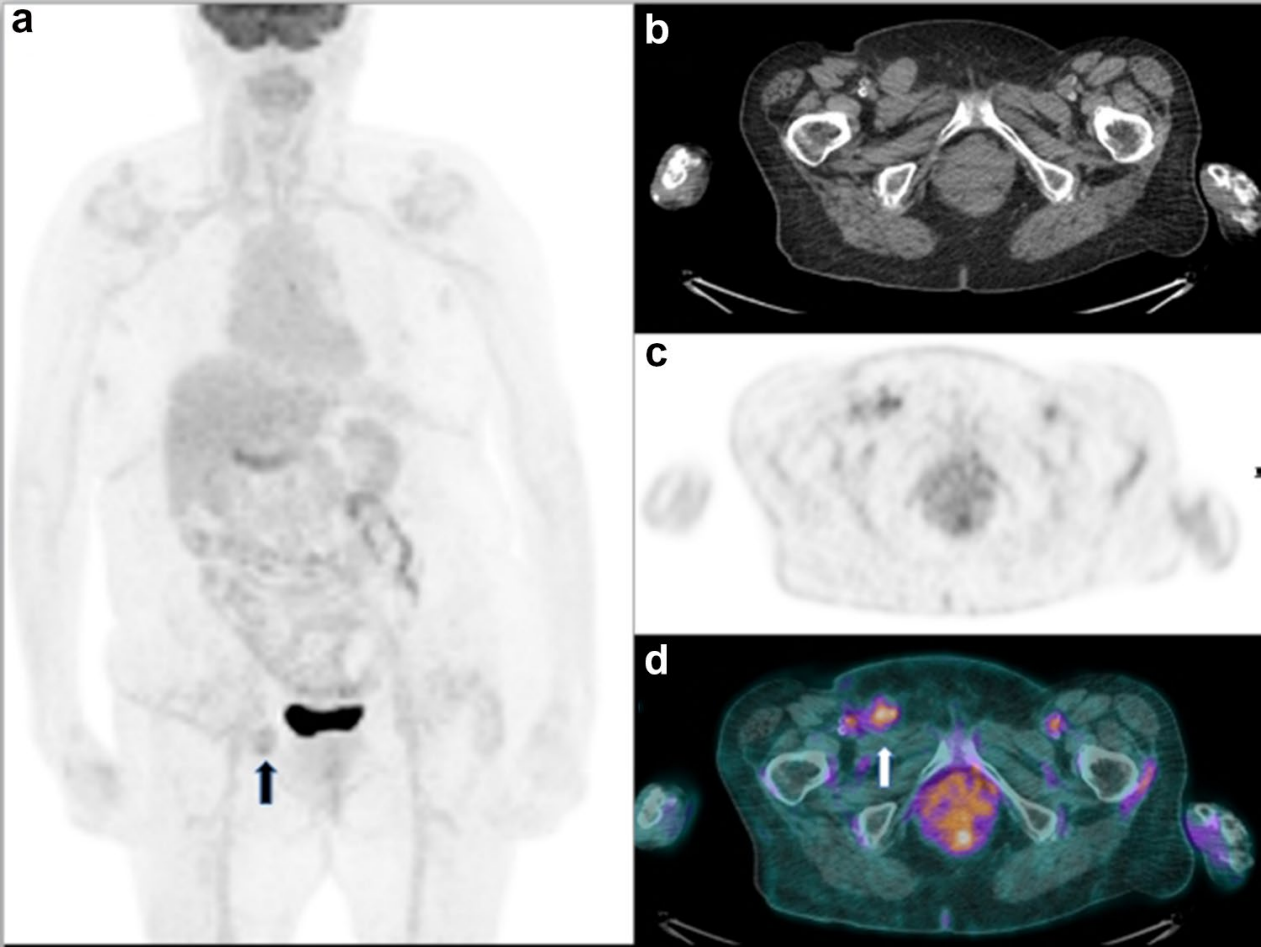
A representative case of false positive ^18^F-FDG PET/CT in 78-year-old woman with a suspected nodal relapse after therapy in a recent pelvic CT. MIP (a) showed the presence of a focal uptake in the right inguinal cave. Axial CT (b), PET (c) and PET/CT fused images (d) confirmed the presence of an increased FDG uptake corresponding to a right inguinal node, but after a specific antibiotic therapy the lymph node disappeared
